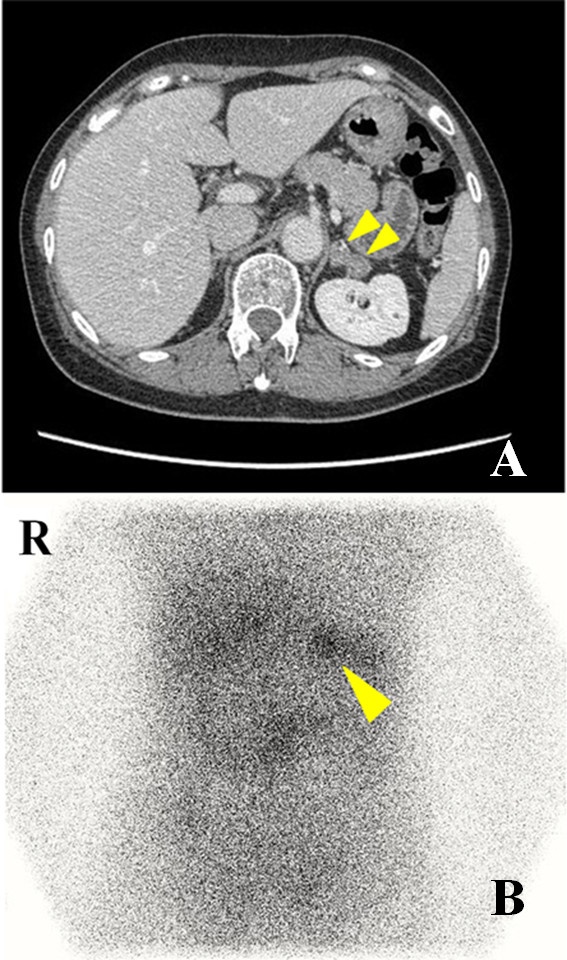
Abdominal computed tomography and scintigraphy for adrenal glands. a: Abdominal computed tomography showed two masses in the left adrenal gland: the diameters of the masses were 12 mm and 9 mm. b: Adrenal scintigraphy revealed tracer accumulation in left adrenal regions.
